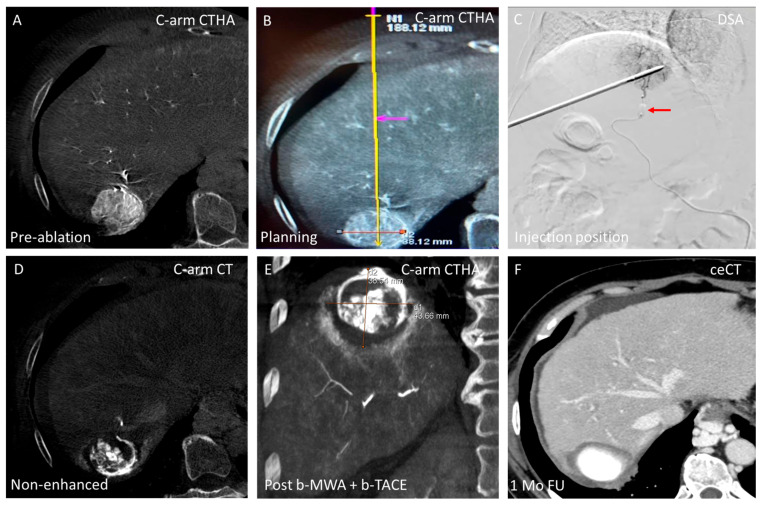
Balloon-occluded MWA followed by balloon-occluded TACE—thermal segmentectomy. (A) Arterial C-arm CT showing a subdiaphragmatic hepatocellular carcinoma (38 mm); (B) Needle trajectory planning using integrated C-arm navigation software (syngo Needle Guidance, Siemens); (C) Digital subtraction angiography (DSA) showing the microwave antenna positioned within the tumor and superselective microcatheter with inflated balloon (Occlusafe, Terumo) (red arrow) occluding arterial flow to the target lesion. Following an arterial stump pressure drop (measured at catheter tip), b-MWA was initiated. Subsequently, b-TACE was performed under continued balloon occlusion using 100 μm drug-eluting microspheres (LifePearl™, Terumo) loaded with 50 mg doxorubicin; (D) Immediately following thermal segmentectomy (b-MWA + b-TACE), a non-enhanced C-arm CT was acquired to identify microbead deposition in the target lesion through contrast entrapped within the beads; (E) Coronal C-arm CTHA (venous phase) confirming complete lesion coverage with adequate ablation margins; and (F) Contrast-enhanced CT (ceCT) at 1-month follow-up (FU) demonstrating complete response.
